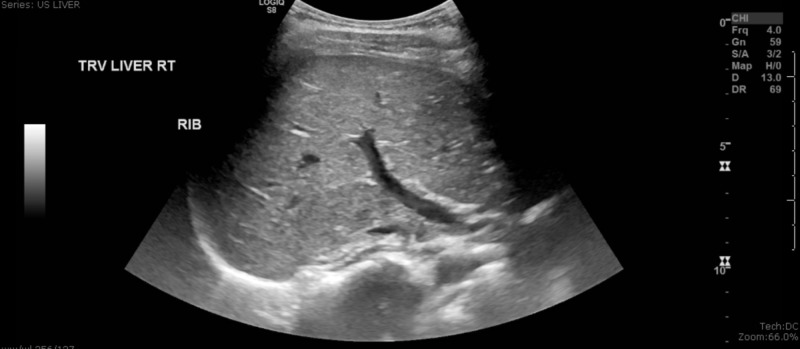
Ultrasound Image of Liver, Right Side Transverse View
